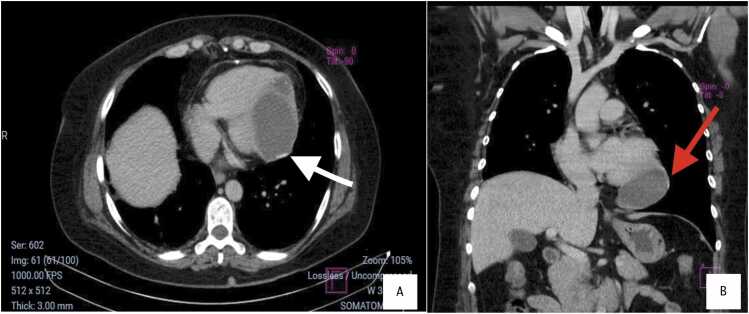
(A) Axial chest CT image showing a multiloculated hypodense mass within the apical region of the left ventricle, with peripheral contrast enhancement and calcifications (95 mm × 47 mm × 68 mm) (white arrow).(B) Coronal reconstruction revealing the full extent of the lesion, with peripheral calcifications (red arrows) and no involvement of the mediastinum.
